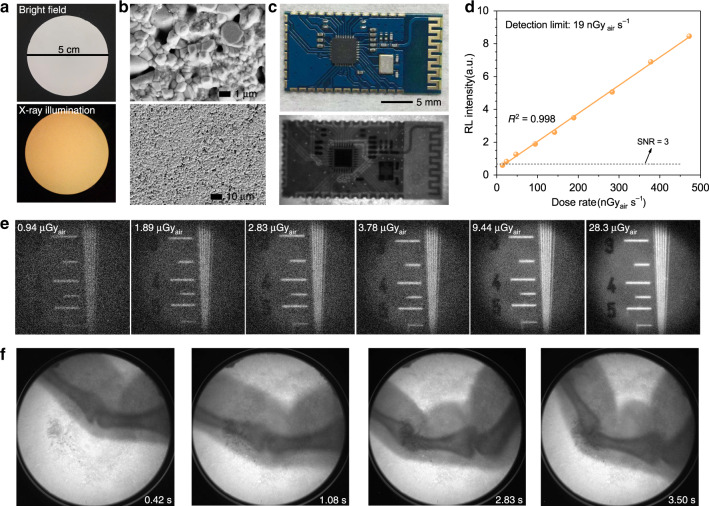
Real-time and low-dose X-ray imaging with Cs_2_Ag_0.6_Na_0.4_In_0.85_Bi_0.15_Cl_6_ scintillator wafers. a Photographs of a Cs_2_Ag_0.6_Na_0.4_In_0.85_Bi_0.15_Cl_6_ wafer under daylight and X-ray illumination (dose rate: 47.2 μGy_air_ s^−1^, voltage: 50 kV). b SEM images of the wafer surface. c Photograph of a circuit board (top) and its X-ray image (below) (dose rate: 47.2 μGy_air_ s^−1^, voltage: 50 kV). d RL intensity of Cs_2_Ag_0.6_Na_0.4_In_0.85_Bi_0.15_Cl_6_ measured at low-dose rates. The detection limit is derived from the fitting curve as the value when the SNR equals 3 (voltage: 50 kV). e X-ray images of the test-pattern plate acquired under different X-ray doses (voltage: 50 kV). f Real-time X-ray images of finger bending (dose rate: 47.2 μGy_air_ s^−1^, voltage: 50 kV)
